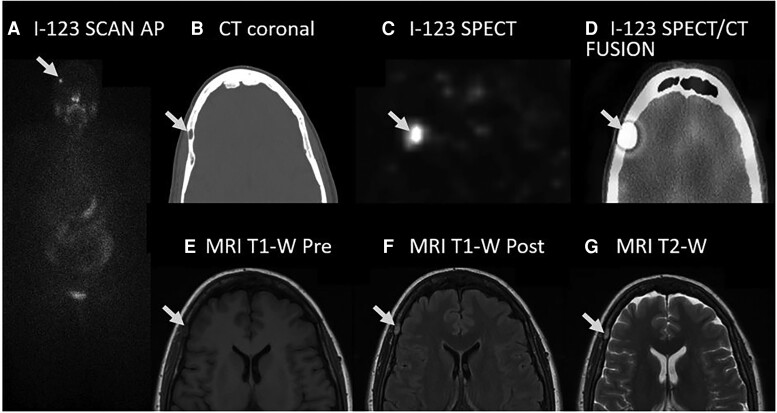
I-123 WBS scan with SPECT/CT imaging and MRI scan of low-risk patient with an interosseus hemangioma (arrow). (A) I-123 WBS anteroposterior (AP) planar image showing iodine trapping in the skull. (B) CT scan, coronal image, without contrast showing a 1.0 × 0.5-cm lytic lesion (arrow) in the right frontal bone. (C) I-123 SPECT scan, coronal image, showing iodine trapping in the right frontal bone. (D) I-123 SPECT/CT fusion scan, coronal image, showing iodine trapping in the lytic lesion (arrow). (E) MRI scan, coronal image, T1-weighted (T1-W) pregadolinium infusion showing hypodense lesion. (F) MRI scan, coronal image, T1-weighted coronal image postgadolinium infusion showing enhancement of the right frontal bone lesion. (G) MRI scan, coronal image, T2-weighted (T2-W) showing enhancement of the right frontal bone lesion.
